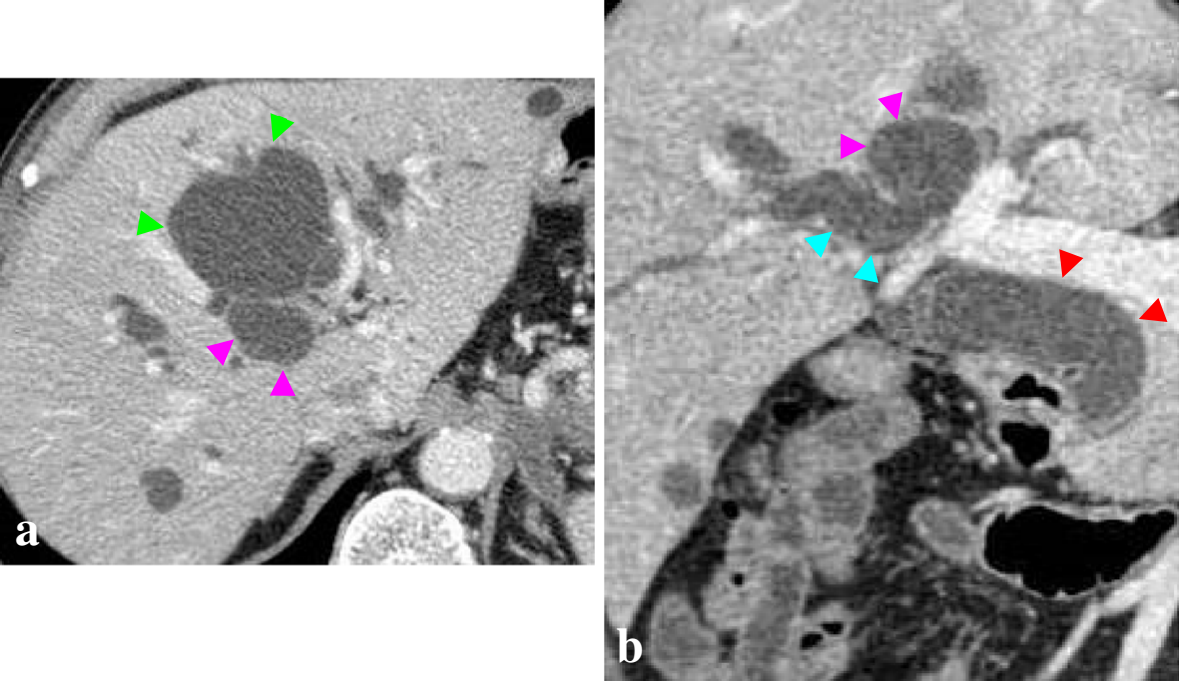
Multidetector row computed tomography. The S1 cyst (*pink arrow head*) connected with the right hepatic duct (*blue arrow head*). The extrahepatic bile ducts were diffusely dilated (*red arrow head*). The tumour mass, stricture, and wall thickness were not evident in either cyst or the entire bile duct. The *green arrow head* shows the S4 cyst. a Axial image. b Coronal image
